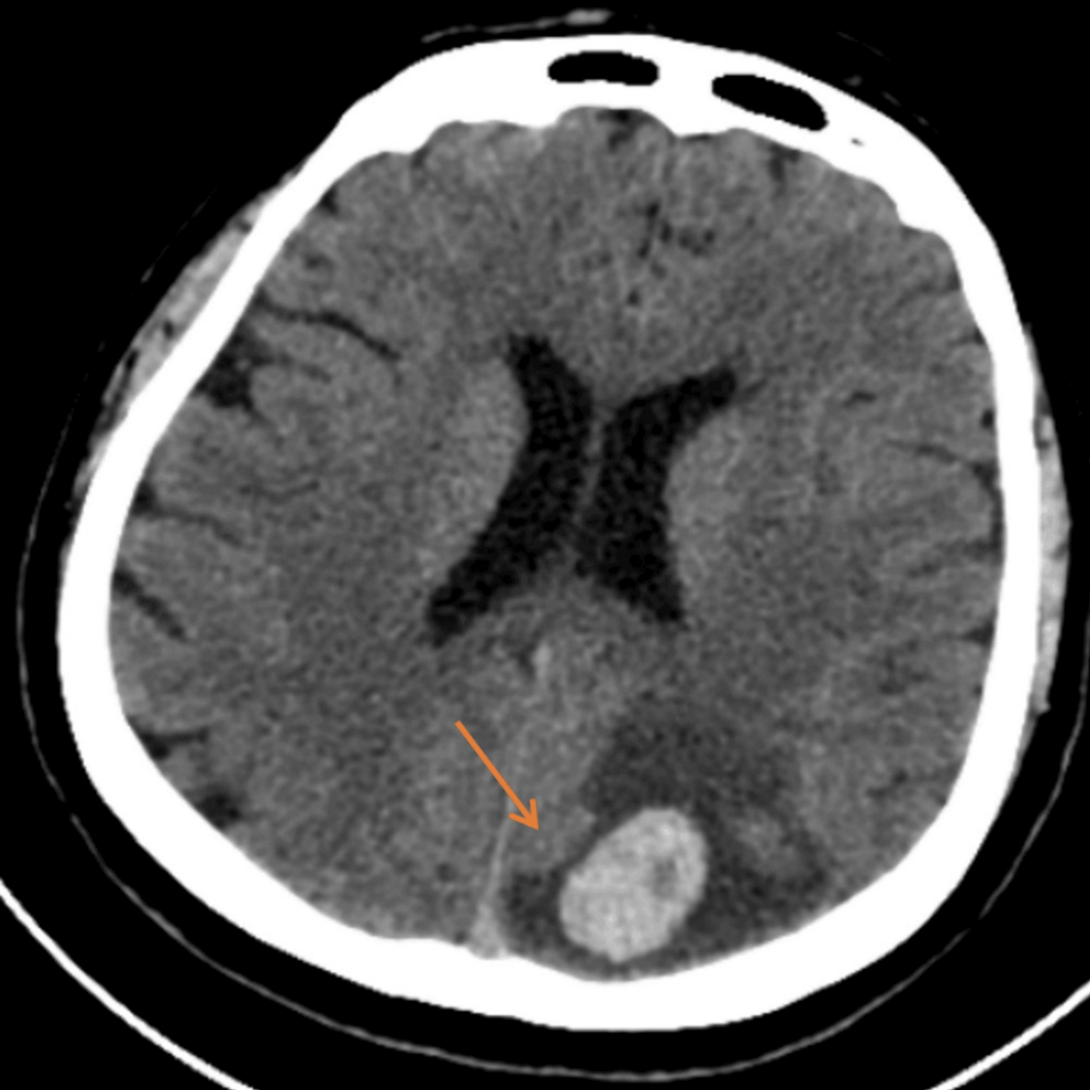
Initial non-enhanced brain CT The axial plane showed a hyperdense lesion at the left parietal lobe surrounded by vasogenic edema with mild effacement of adjacent sulci. Conclusion of the first CT: This was assumed to be an intraparenchymal hemorrhage or hemorrhagic lesion such as a ruptured cavernoma.
